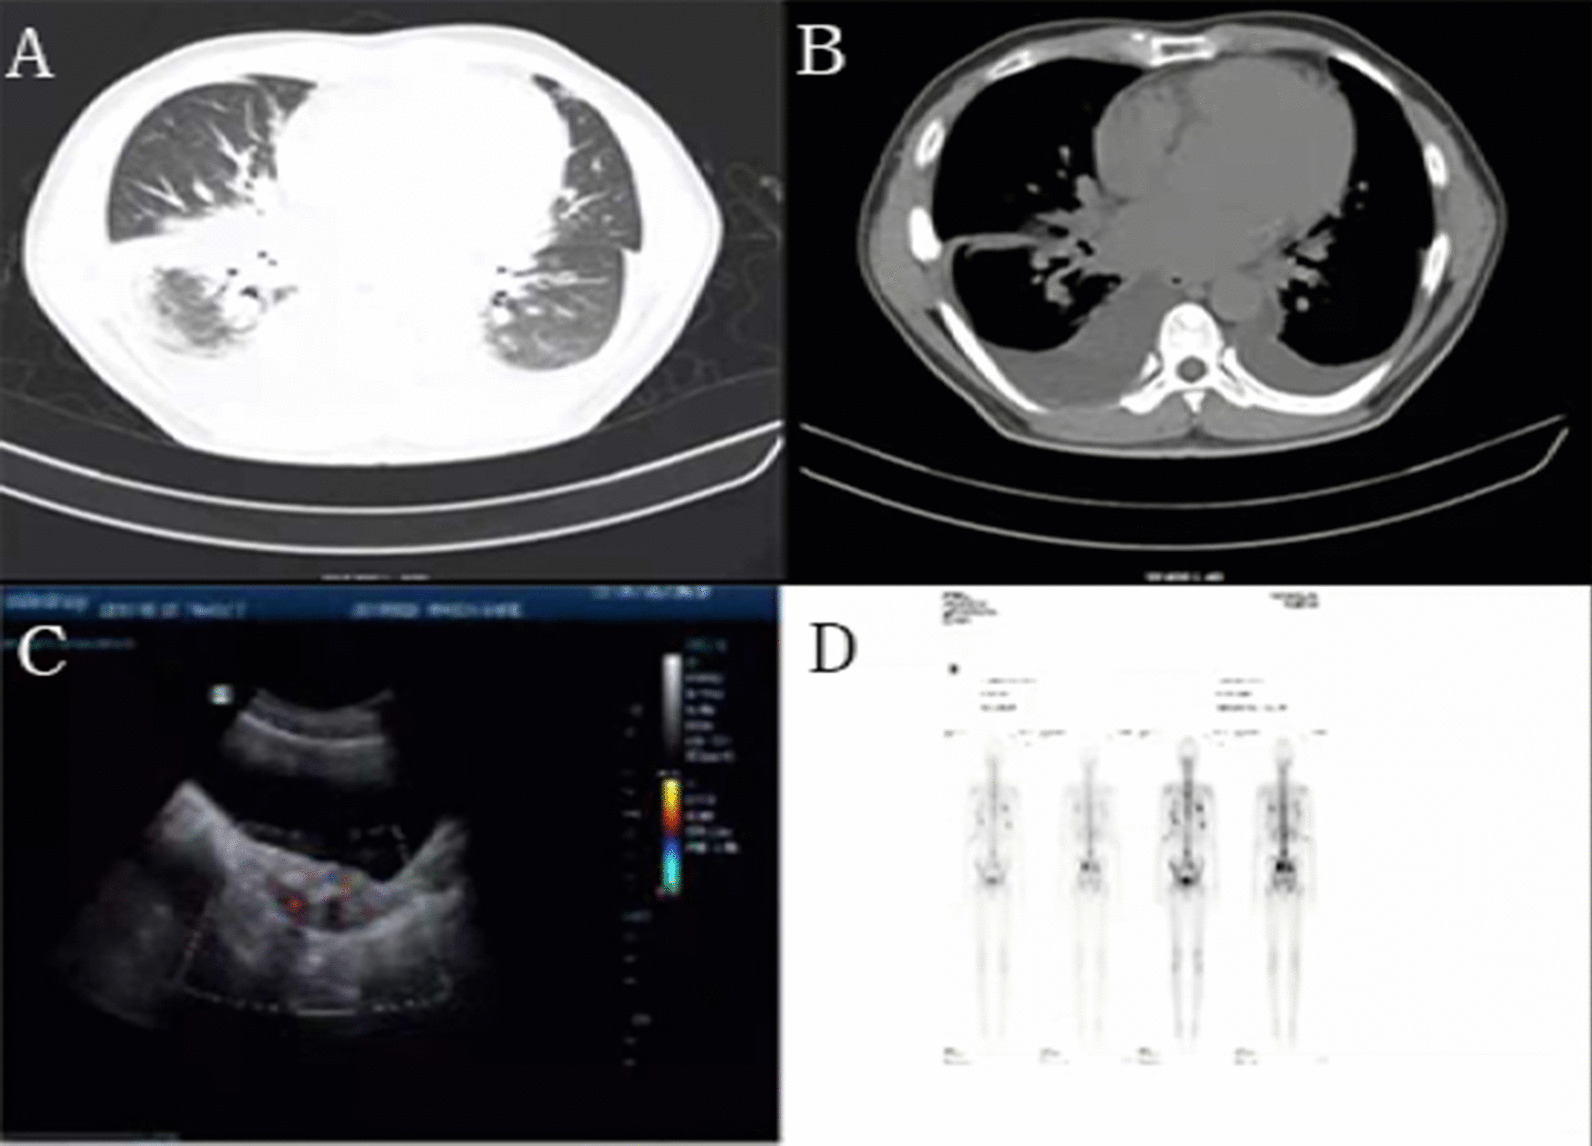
A and B Chest computed tomography showing bilateral pleural cavity and right interlobar fissure effusion. C Renal ureteral ultrasound showing pelvic hypoecho. D Bone ECT (Emission Computed Tomography) showing multiple bone metastases throughout the body
